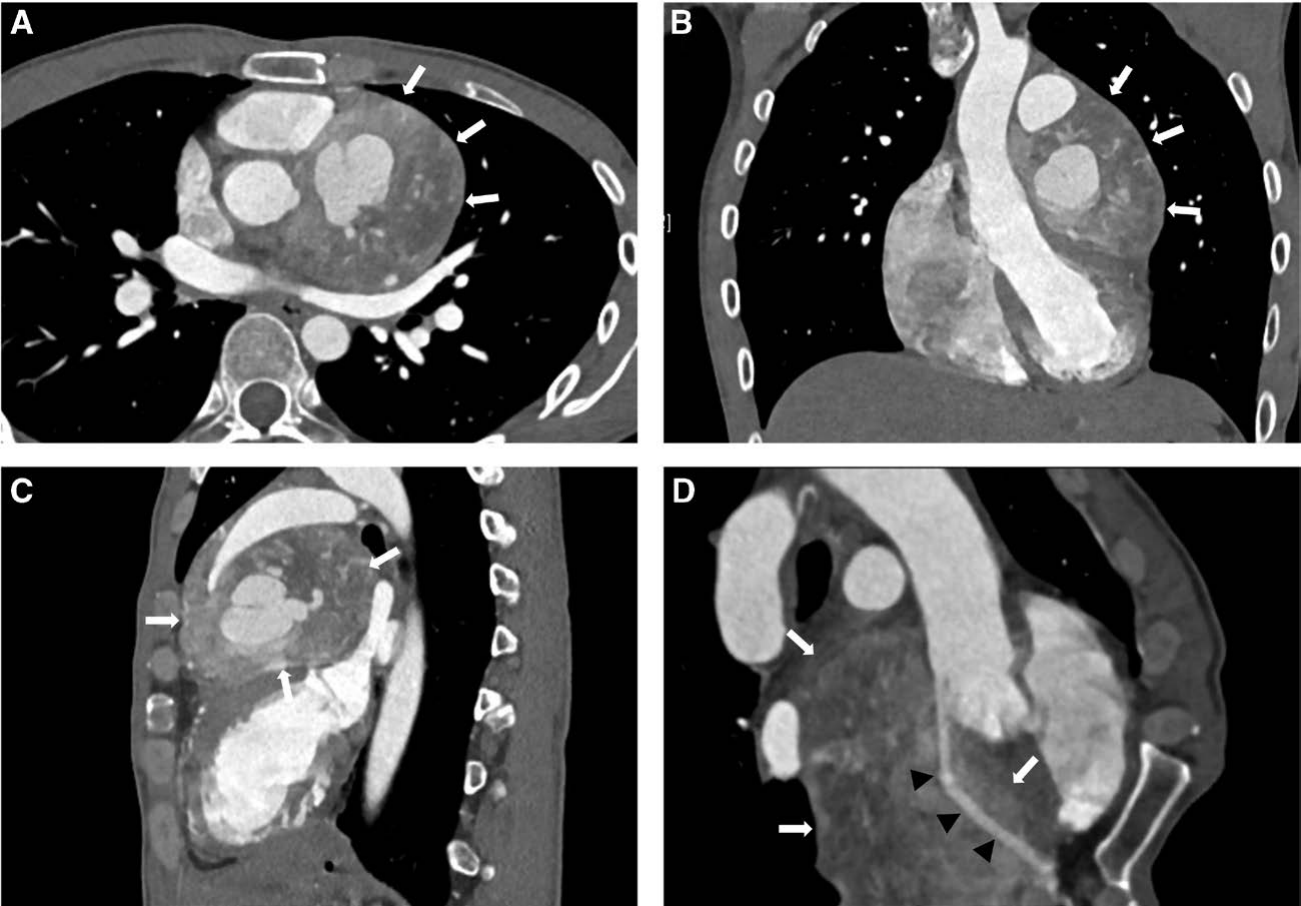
Axial (A), coronal (B) and sagittal (C) contrast-enhanced computed tomography images showed a hypervascularized heterogenous mass in the middle mediastinum (arrows). A magnified multiplanar curved reformat view of the proximal left anterior descending artery (arrowheads) showed that it was enclosed by the tumor (arrows), but the lumen was patent (D).
